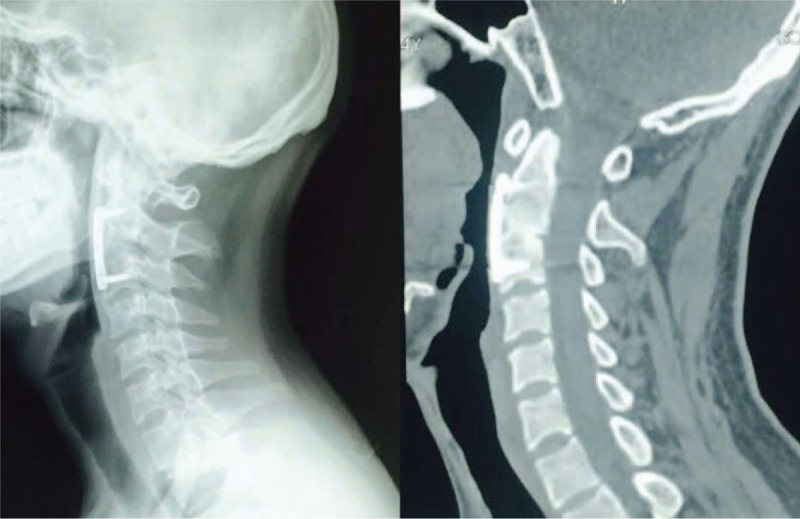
Three months postoperative x-ray and CT scan showed a good position of the implant and bony fusion at the C2/3 segment. CT = computed tomography.
